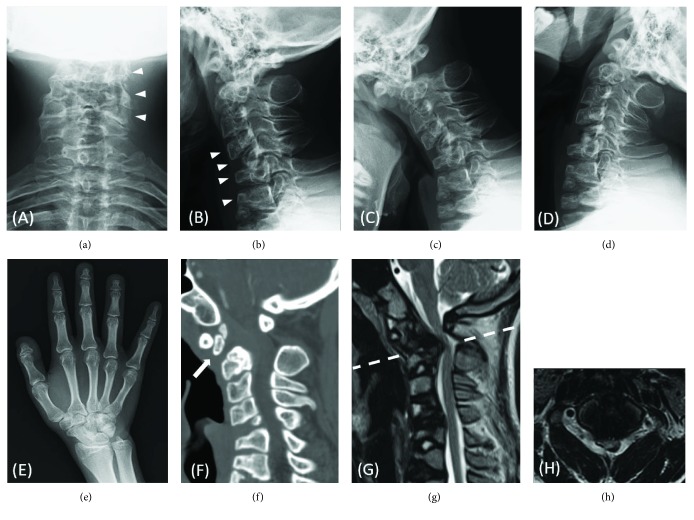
(a, b) Preoperative anterior-posterior and lateral plain radiographs of the cervical spine revealing multiple dysplastic vertebral bodies (*white arrowheads*). (c, d) Preoperative lateral plain radiographs of the cervical spine clearly demonstrating unstable atlantoaxial subluxation. (e) An anterior-posterior plain radiograph of the right hand revealing typical shortening of the distal phalanges (brachytelephalangy) of the thumb and middle and ring fingers. (f) Preoperative sagittal reconstructed computed tomography showing the segmented os odontoideum (*white arrow*). (g) Preoperative sagittal T2-weighted magnetic resonance (MR) images showing a severely compressed spinal cord at the C1/2 level with signs of myelomalacia inside the cord. A low-intensity retroodontoid mass is evident on the T2-weighted MR images. (h) Preoperative axial T2-weighted MR image at the C1/2 level revealing a severely compressed spinal cord. The white dotted lines in (g) indicate the level of (h).
